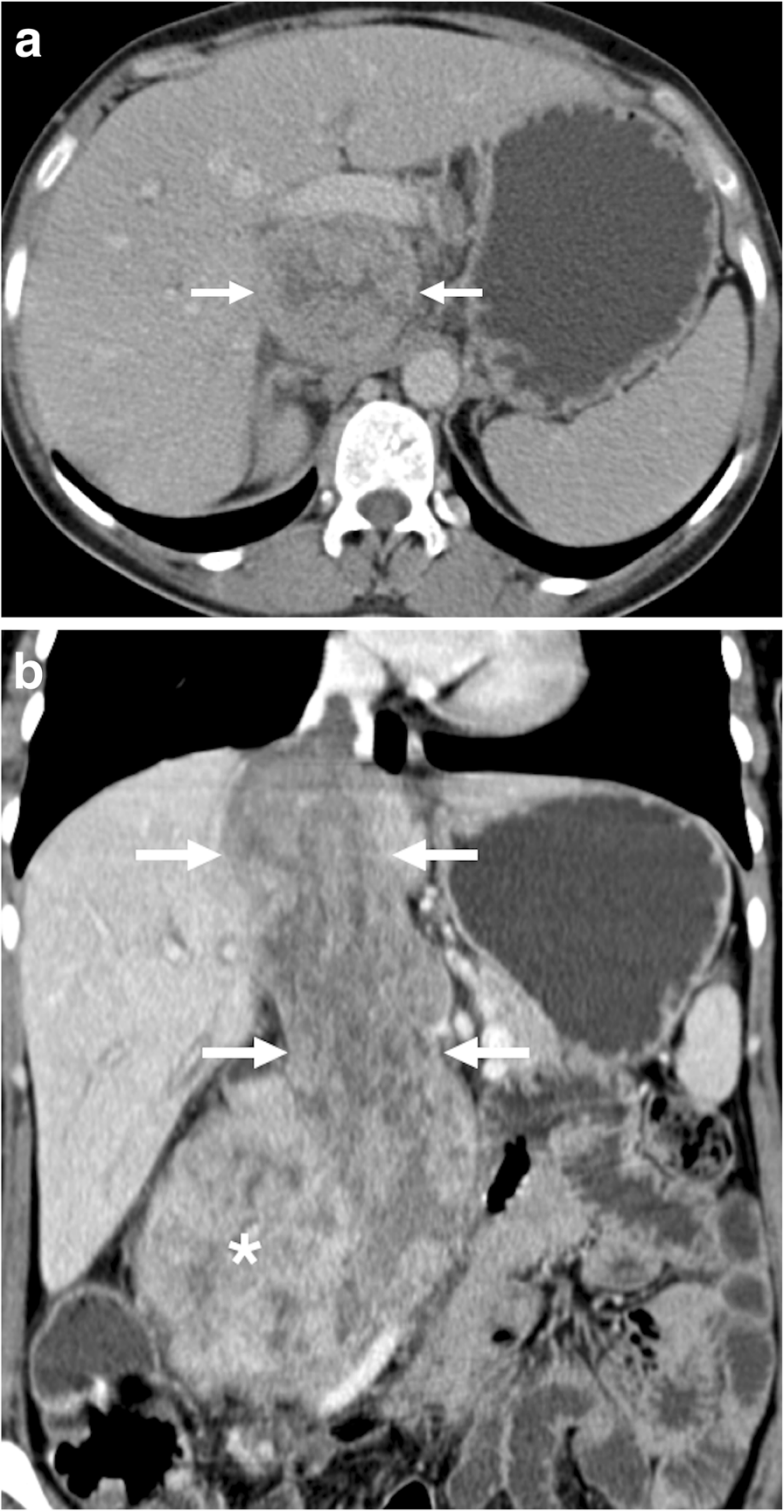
A 35-year-old female with no significant past medical history presented with palpable mass in the abdomen and bilateral leg swelling. a Axial plane postcontrast CT image shows a contrast enhancing expansile mass within the IVC lumen (arrows). b Coronally reformatted postcontrast CT image shows the extension of the tumor along the trajectory of the IVC (arrows) with prominent extraluminal extension (asterisk). Biopsy confirmed leiomyosarcoma of the IVC
